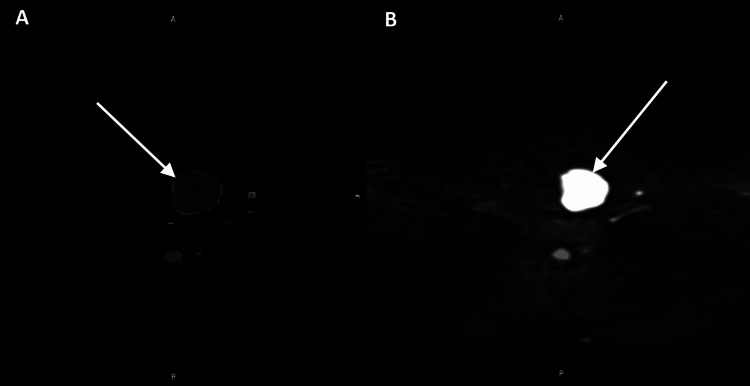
Diffusion-weighted imaging (DWI) (A) and apparent diffusion coefficient (ADC) map (B). The lesion exhibits restricted diffusion (arrows), indicating viscous/proteinaceous content. This key finding explains the repeated nondiagnostic (Bethesda I) FNAB results. FNAB: Fine-needle aspiration biopsy
